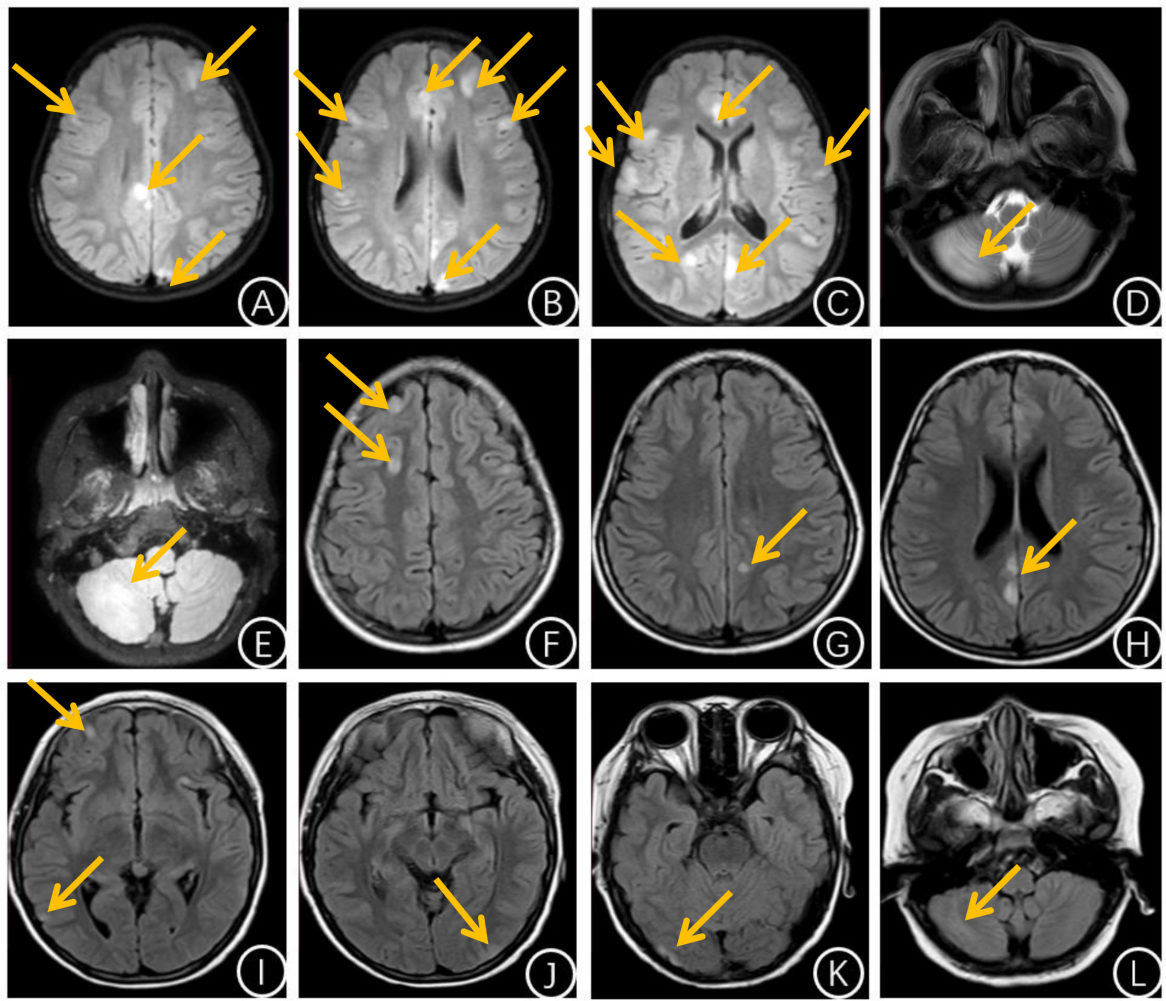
(A-C) Brain MRI-FLAIR during the initial acute phase of the child’s illness revealed multiple patchy areas of hyperintensity in the bilateral frontal, parietal, temporal, and occipital regions. Most of these lesions were located in the cortical areas and showed no significant enhancement post-contrast. (D, E) Brain MRI during the second acute episode revealed a large patchy area of hyperintensity in the right cerebellar hemisphere on both T2-weighted and FLAIR sequences. (F-L) Brain MRI-FLAIR during the third acute episode revealed hyperintensities in the bilateral frontal, temporal, occipital, and parietal lobes, the cingulate gyri, and the right cerebellar hemisphere. The bilateral hippocampi appeared to be involved.
